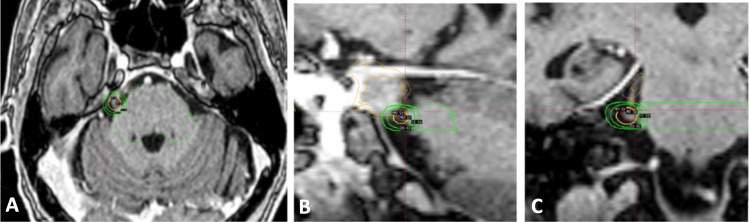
Views of gamma knife radiosurgery plan targeting the right trigeminal nerve Representative (A) axial, (B) sagittal, and (C) coronal T1-weighted contrast-enhanced MR image of single fraction gamma knife radiosurgery plan targeting the patient’s right trigeminal nerve. Yellow = 50% (40 Gy) isodose line, green = 20 Gy and 12 Gy isodose line, light green = brainstem, red = right trigeminal nerve, orange = meningioma + 1 mm margin.
